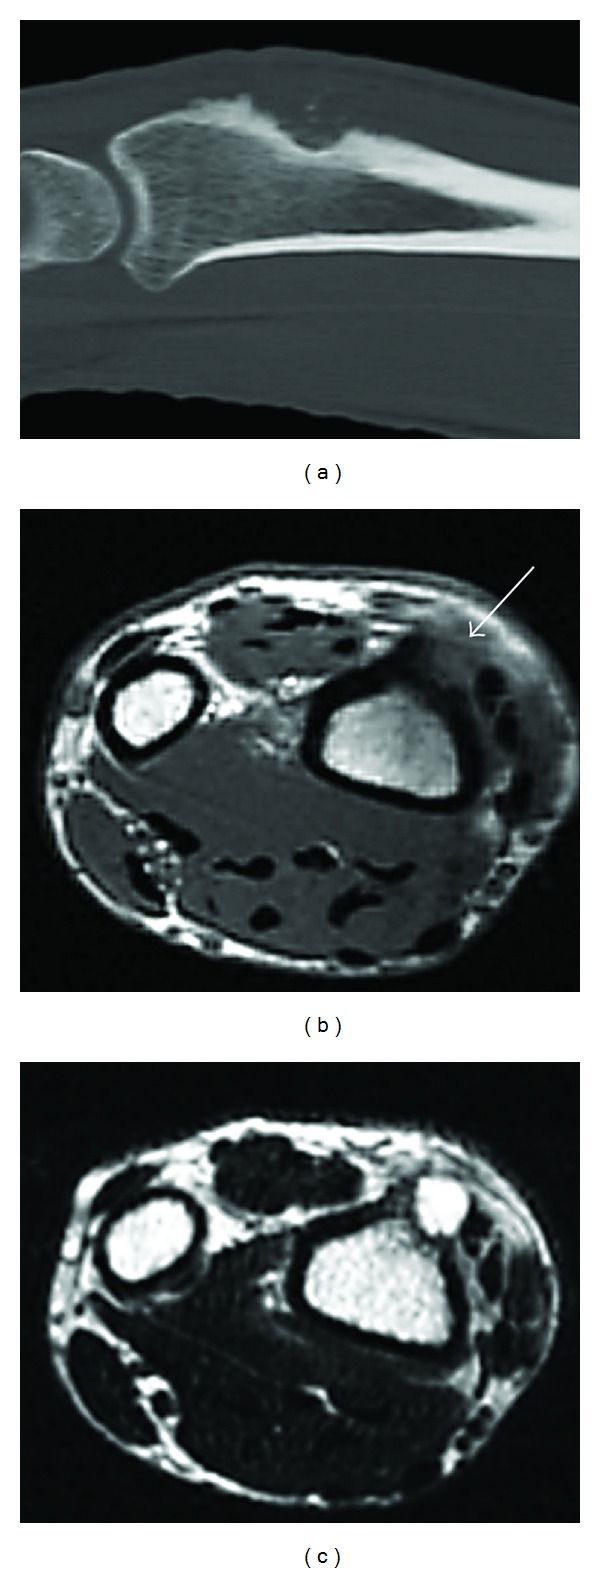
Imaging studies. (a) Sagittal CT-scan showing a lytic lesion on the dorsal cortex of the distal radius containing punctiform calcifications. ((b)-(c)) Axial MR images showing a soft tissue component (arrow) associated with the cortical lesion. Its appearance is isointense on T1 (b) and hyperintense on T2-weighted images (c).
